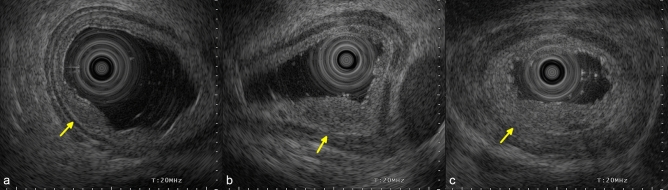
Endoscopic ultrasonography findings of local residual/recurrent cancer after CRT/RT. (a) Uninterrupted fifth layer (arrow). (b) Slurred fifth layer (arrow). (c) Ruptured fifth layer (arrow). CRT, chemoradiotherapy; RT, radiotherapy.
